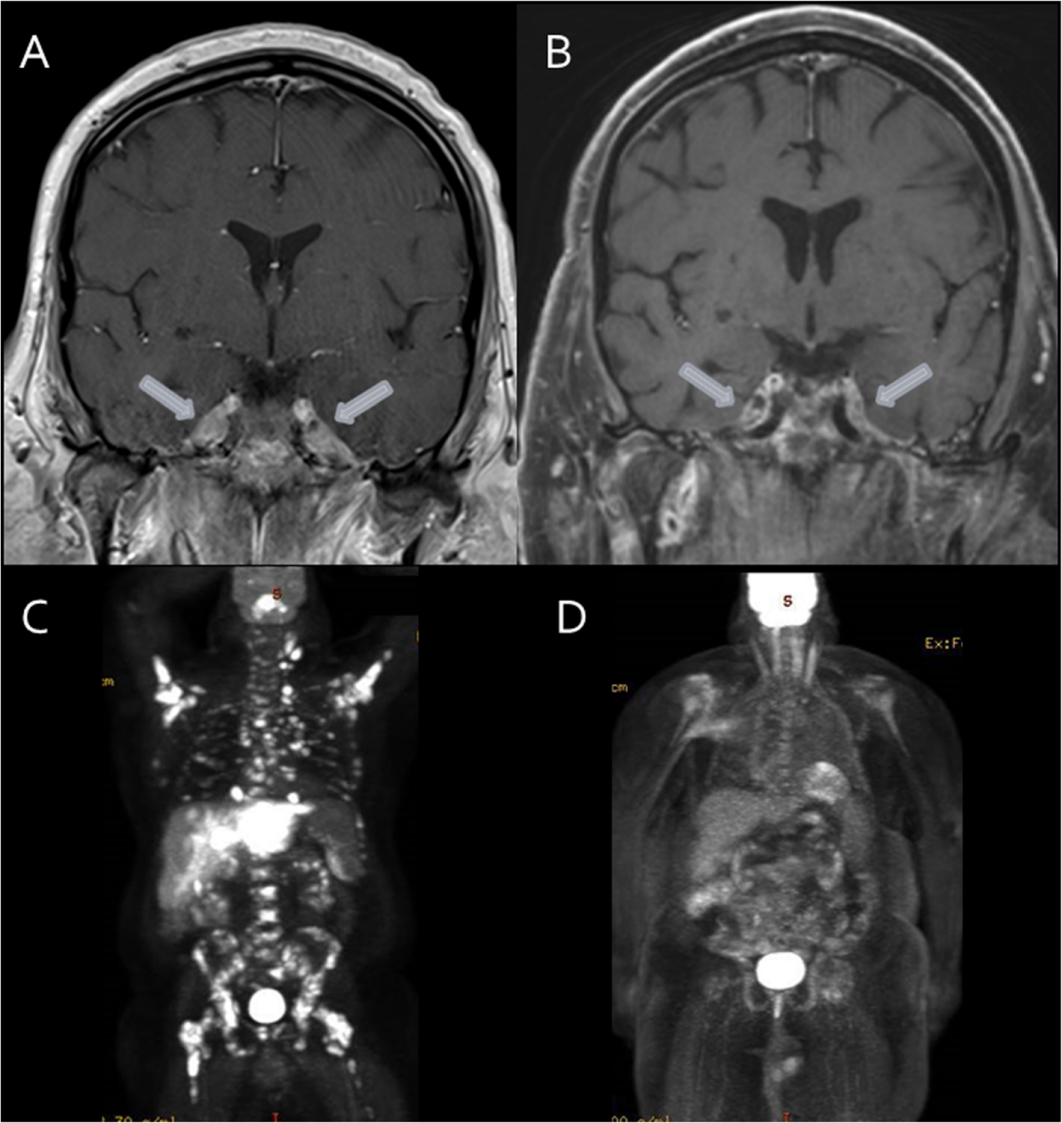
Diffuse large B cell lymphoma involvement on magnetic resonance imaging and positron emission tomography-computed tomography. Magnetic resonance images of brain show: a abnormal enhancement of Meckel’s cave bilaterally with extension through the foramen ovale (arrows) before chemotherapy and b decreased soft tissue in Meckel’s cave bilaterally (arrows) after chemotherapy. Positron emission tomography-computed tomography scans show: c extensive hypermetabolic lesions throughout the axial and appendicular skeleton, including the skull base, as well as fluorodeoxyglucose-avid lymph nodes above and below the diaphragm before chemotherapy and d marked response of all hypermetabolic lesions after chemotherapy
